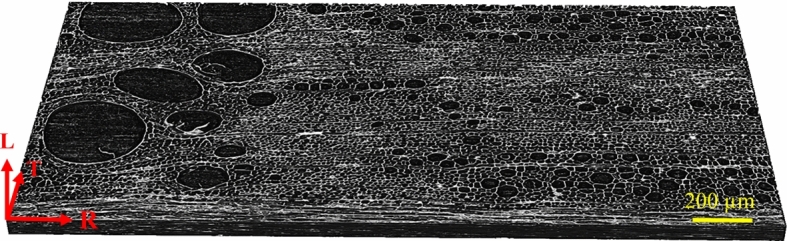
Image of an oak wood micro-structure containing a single growth ring and a horizontal band of multi-seriate rays (located at the bottom edge of the micro-structural region), as obtained from $$\mu$$CT scanning. The original contrast of the scanned greyscale image has been increased in this figure to enlarge the clarity of micro-structural details (colour figure online)
